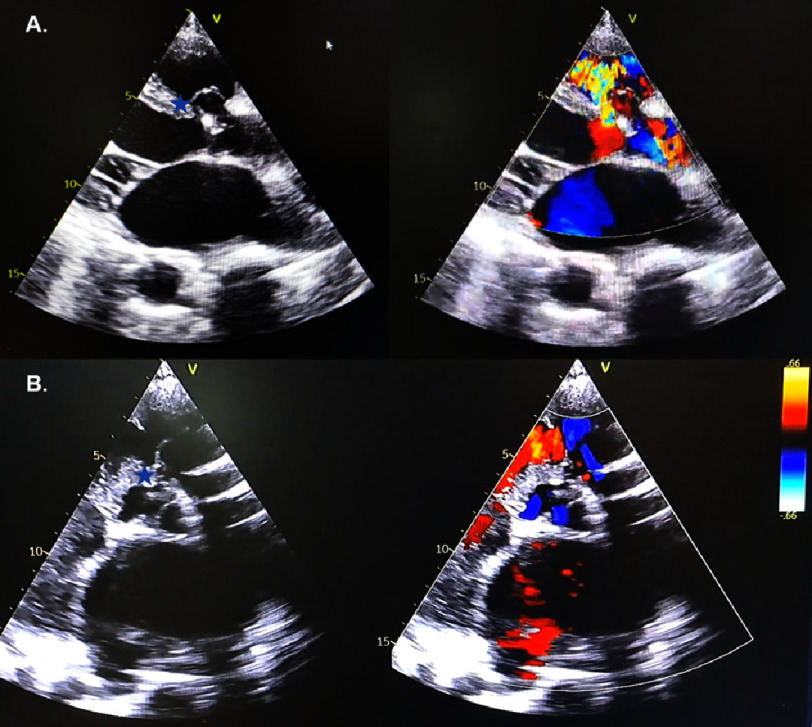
Echocardiography showing (A) PLAX view; (B) PSAX view. Blue star indicates interventricular septum gap with size 0.3 mm with shunt left to right).
